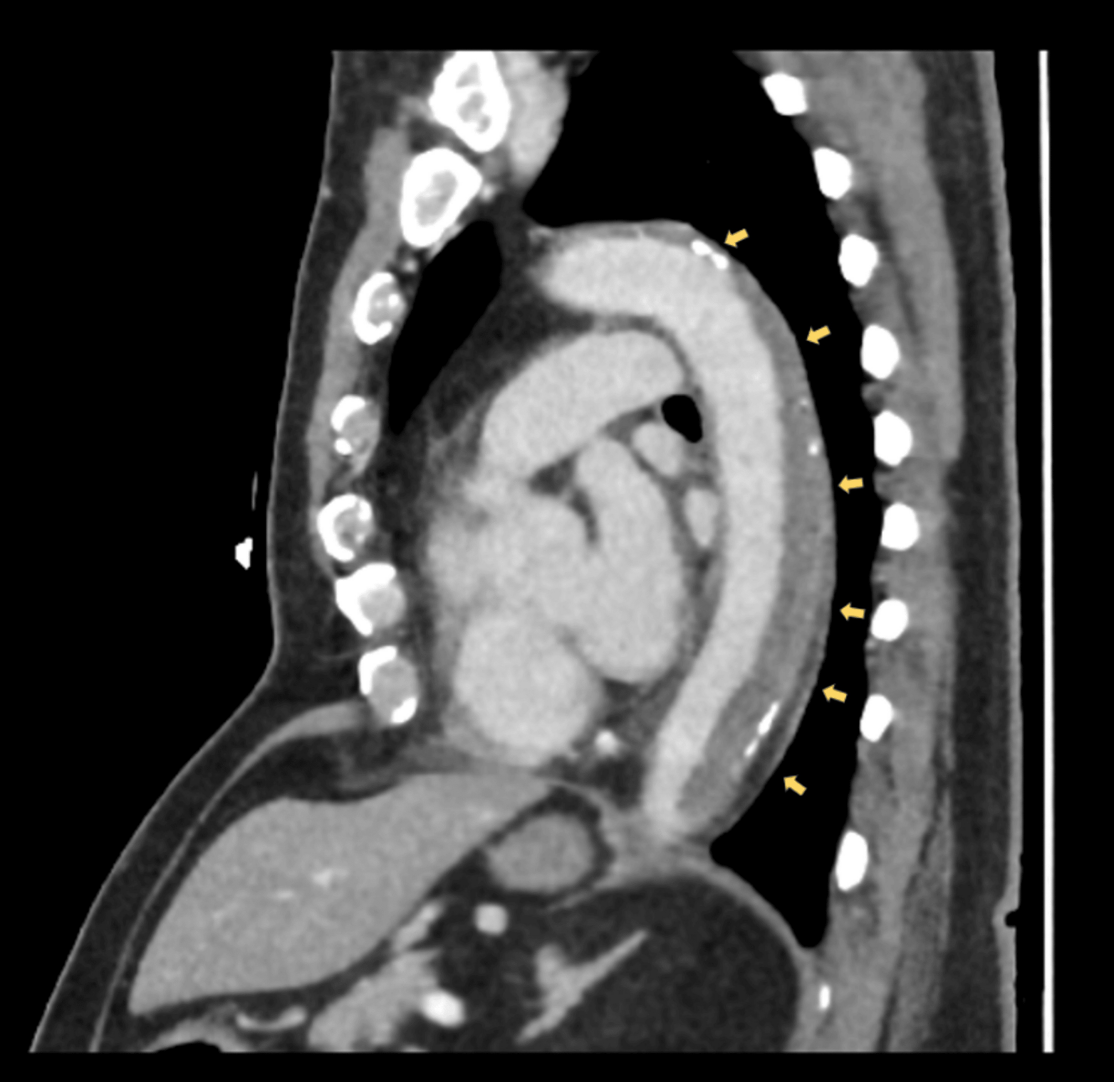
Sagittal CT angiography of the thoracic aorta. Demonstrates mural thrombus along the posterior wall of the descending thoracic aorta (yellow arrows), occupying approximately 50% of the aortic lumen. CT: computed tomography
